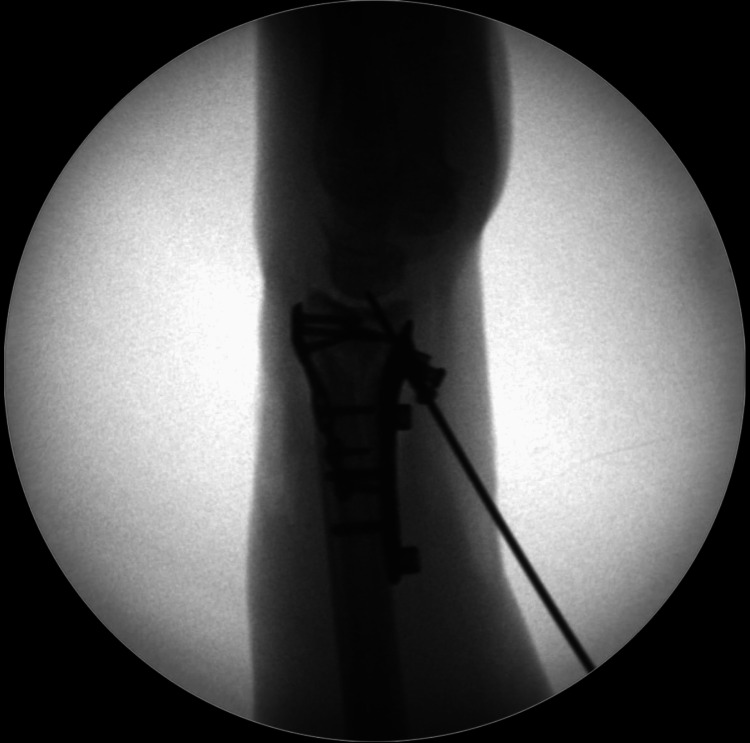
Lateral intraoperative radiograph of combined volar and dorsal plate fixation Although rarely required, a combined collar and dorsal approach and fixation is required. Here, the dorsal fragment would not fully reduce with closed methods, as there was incarceration of an extensor tendon between the dorsal fragment and the distal radius.
